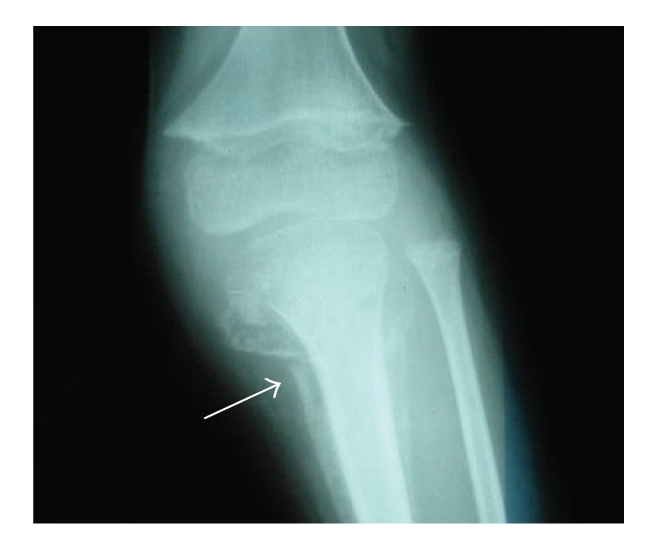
Radiograph of knee showing: an irregular thickened white line at the metaphysis of the femur, a small beak-like excrescences at the metaphysis of the femur, and a detachment of the periosteum of the tibia.
